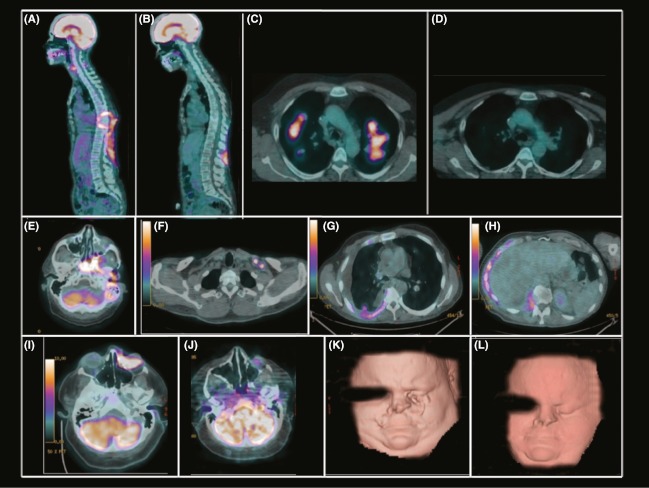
Representative images from 18-FDG PET/CT scans. Panels A–D represent baseline and follow-up 18F-FDG PET/CT scans in two separate subjects with soft tissue, bone, and muscle involvement (panels A and B; SUVmax 5.6 vs. 2.8 baseline vs. follow-up, respectively) and lung involvement (panels C and D; SUVmax 12.6 vs. 0.2 at baseline vs. follow-up, respectively). Panels E–H are sample images demonstrating typical locations of BCC metastasis on 18-FDG PET/CT in the skull base (E), lymph nodes (F), lungs, bone, and pleural space (G and H). Panels I–J show the value of baseline axial 18-FDG PET/CT image and three-dimensional CT volume rendering depicting the extent of superficial and deep tissue lesion involvement of a hypermetabolic lesion (SUVmax 21.1; panels I and K) of the inferior left orbit, left cheek, nasal tissues, and maxillary tissue. After therapy, the axial 18-FDG PET/CT fusion image and three-dimensional CT volume rendering exhibited significant reduction in metabolic activity (SUVmax <2.0) and soft-tissue healing (panels J and L).
